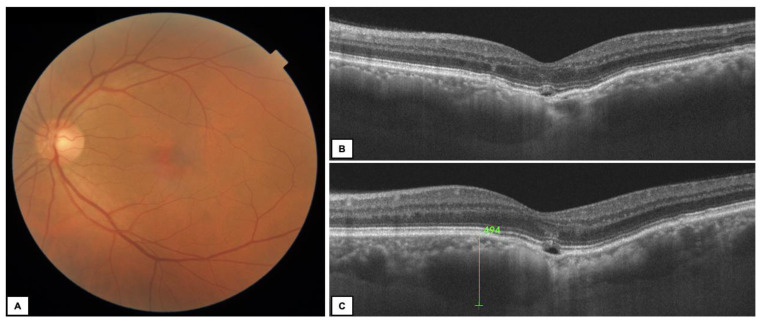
A 64-year-old man with subfoveal FCE type 2 in the left eye. (A) Color fundus photography—subtle alteration of the RPE in the fovea; (B,C) OCT B-scans—non-conforming FCE with increased CT (494 μm) and pachyvessels nasally to the lesion (C).
